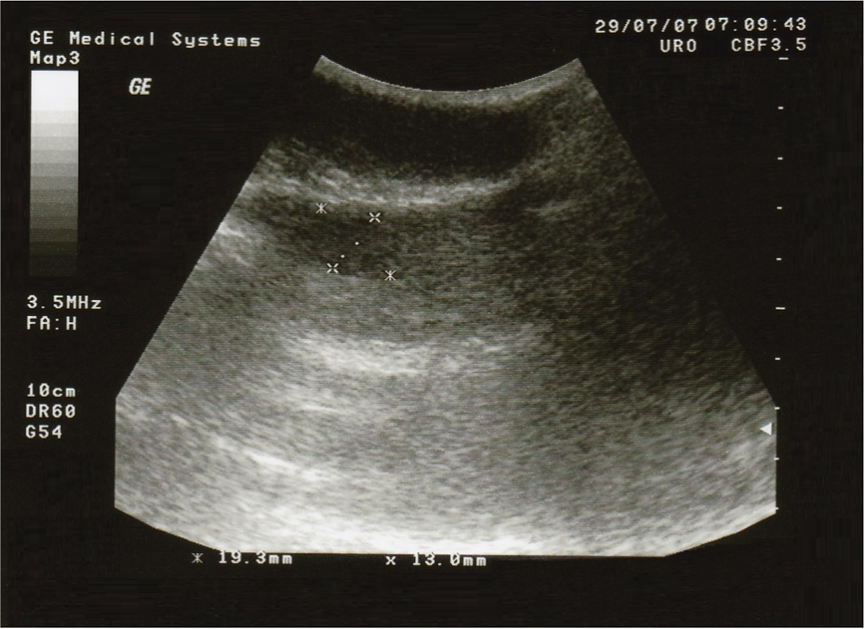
Ultrasound of a right testis with Leydig cell tumor. The tumor appears as a hypoechoic non-homogeneous mass within the testicular parenchyma.
